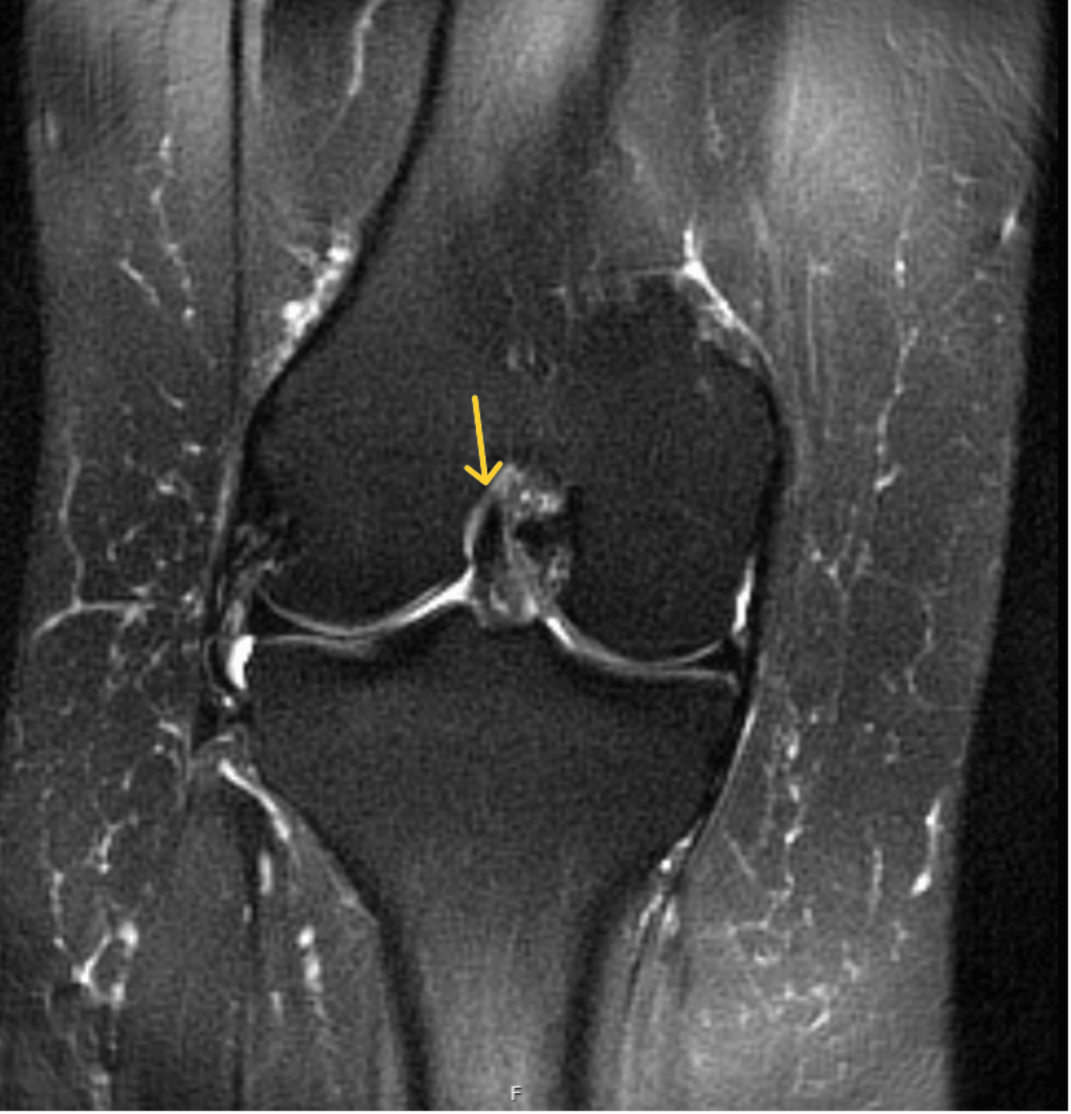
Coronal T2-weighted MRI of the right knee demonstrating an intact ACL (yellow arrow) ACL: anterior cruciate ligament
